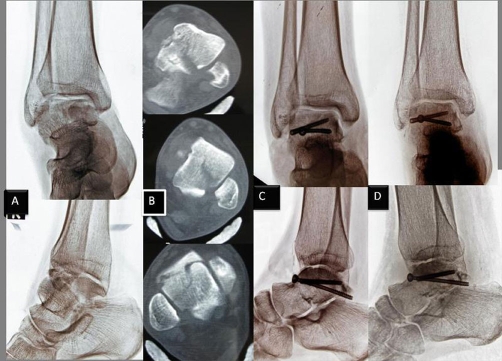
Preoperative and postoperative radiological datas of case 2. Preoperative anteroposterior and lateral plain radiographs (A). CT scans demonstrated a talar body fracture lines associated with comminution (B). The patient underwent open reduction and internal fixation through anterolateral approaches (C). 12 months later, the fracture had united with a congruent ankle joint and without evidence of osteonecrosis (D).
